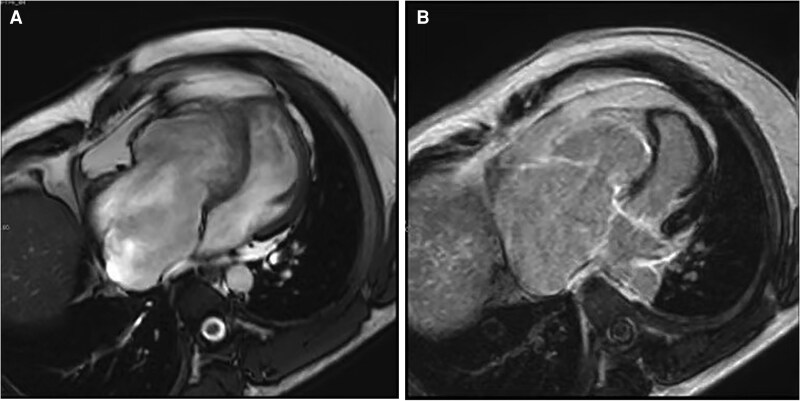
The cardiac magnetic resonance imaging revealed dilated right heart (*A*) and endocardial late gadolinium enhancement in right ventricular (*B*).
